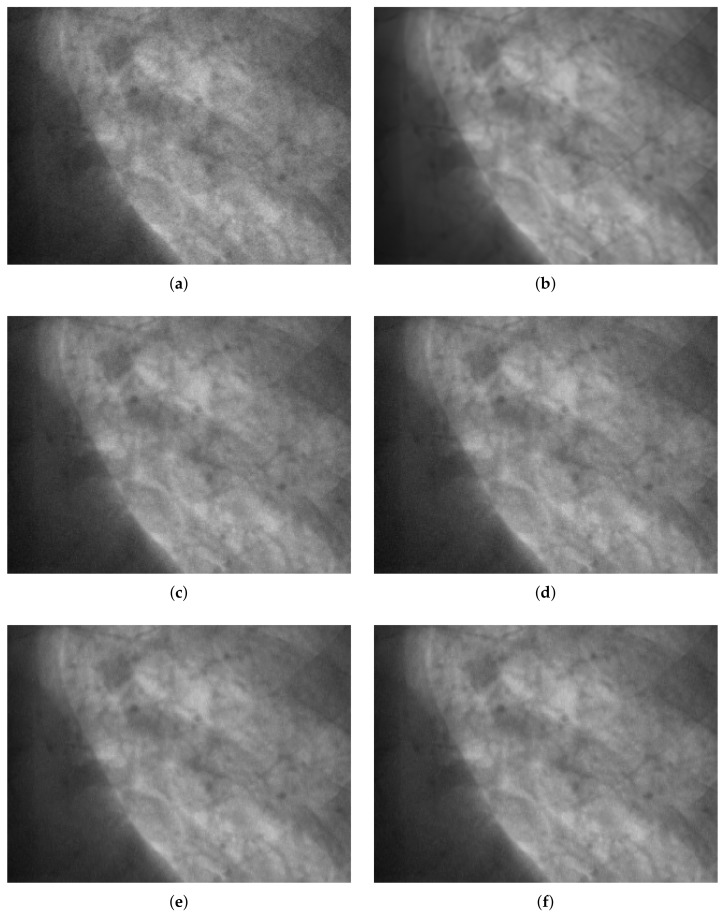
Partially magnification of Figure 16. (a) low-dose X-ray noisy image; (b) noiseless image; (c)–(f) denoising results; (c) Du’s method [29] + hard threshold; (d) proposed method + hard threshold; (e) Du’s method [29] + soft threshold; (f) proposed method + soft threshold.
